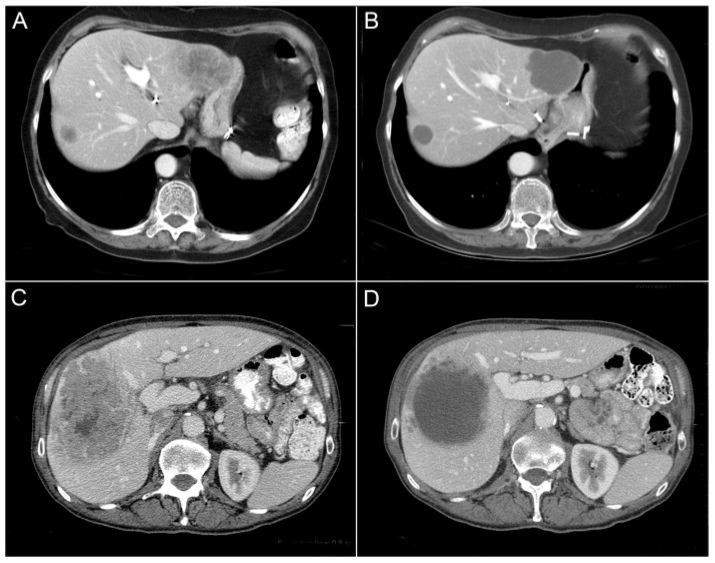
Pre- and post-treatment CT scans showing RECIST stable disease and morphologic optimal response (A,B), characterized by homogeneous attenuation and sharp tumor–liver interface; morphologic incomplete response (C,D), with homogeneous attenuation but ill-defined tumor–liver interface remaining after treatment. Adapted from Chun et al. [10] with permission.
